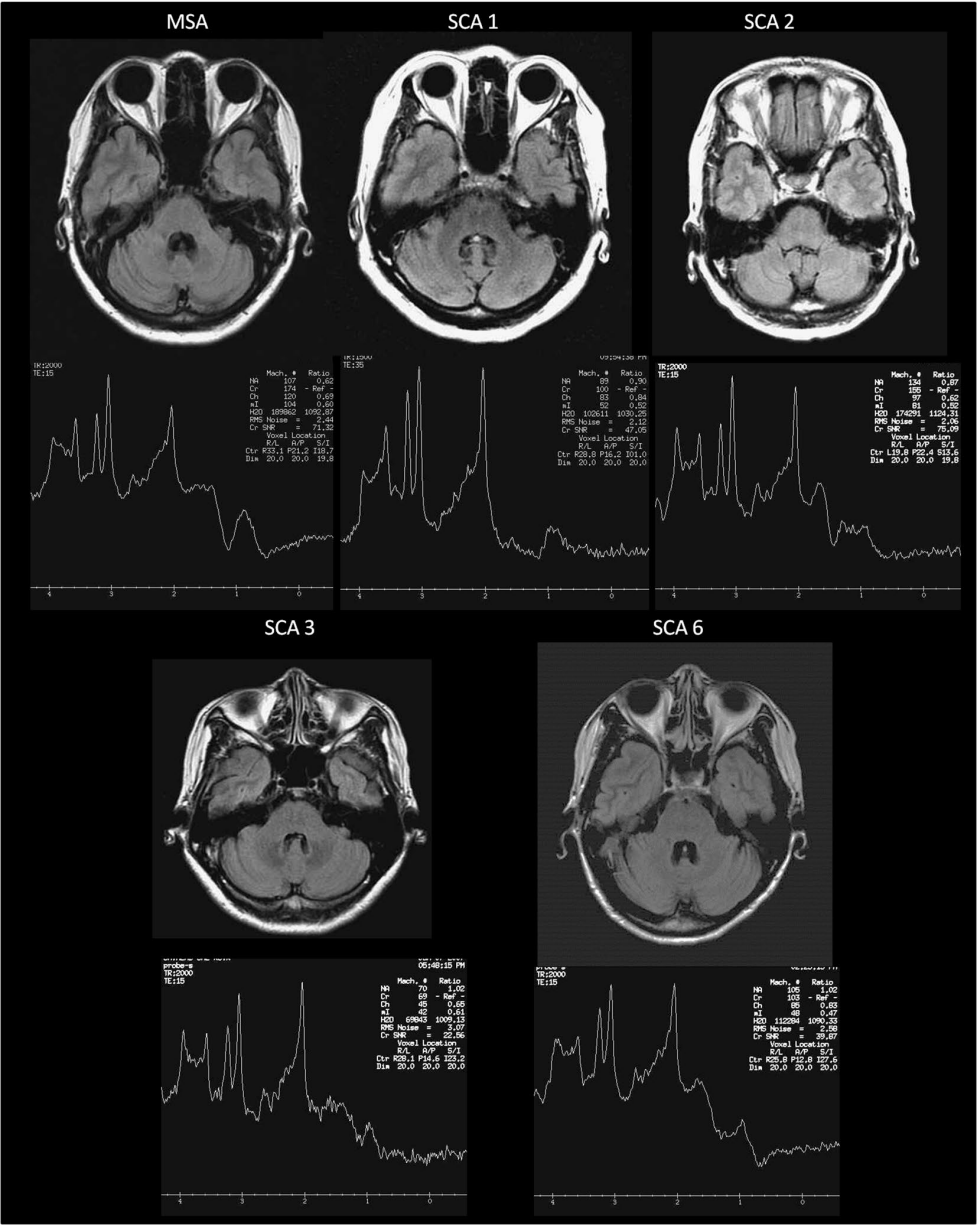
Axial FLAIR images at pons level and cerebellar MRS of patients with MSA or SCAs.
